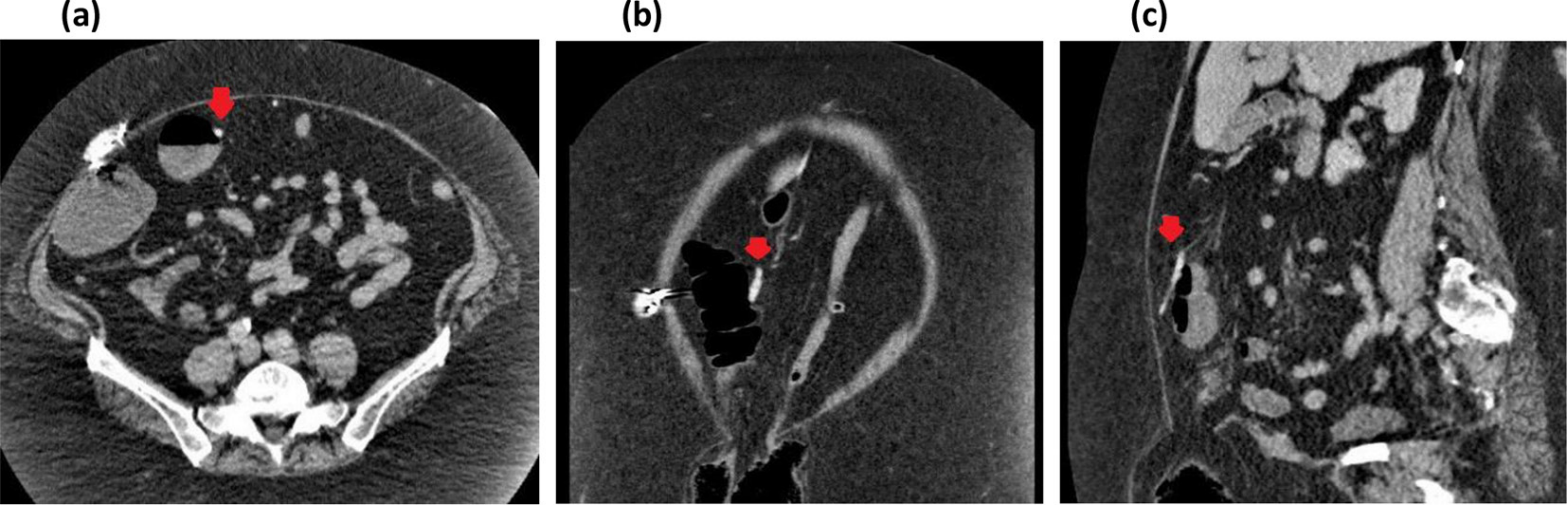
Axial (a), coronal (b) and sagittal (c) CT images showing the position of the laparoscopic-adjustable gastric band tubing (red arrow) adjacent to the transverse colon at time of initial presentation to our facility, 18 months prior to intracolonic penetration of the tubing.
